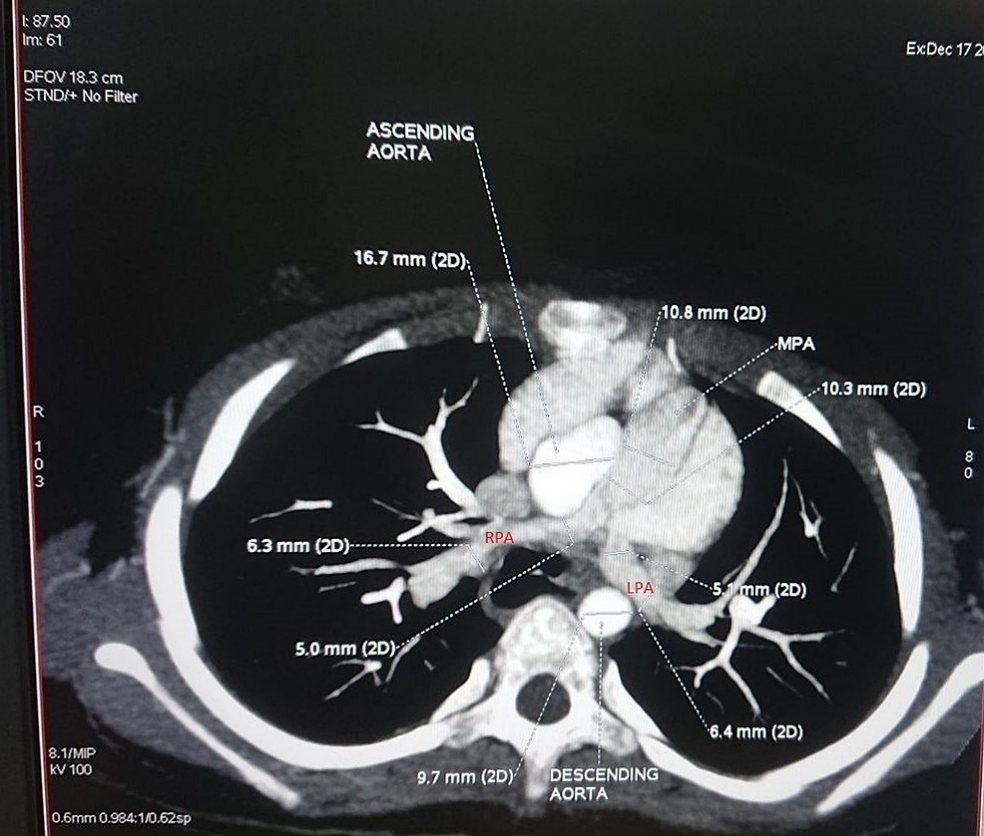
Right and left pulmonary artery stenosis depicted in the cardiac CT scan. CT: computed tomography
